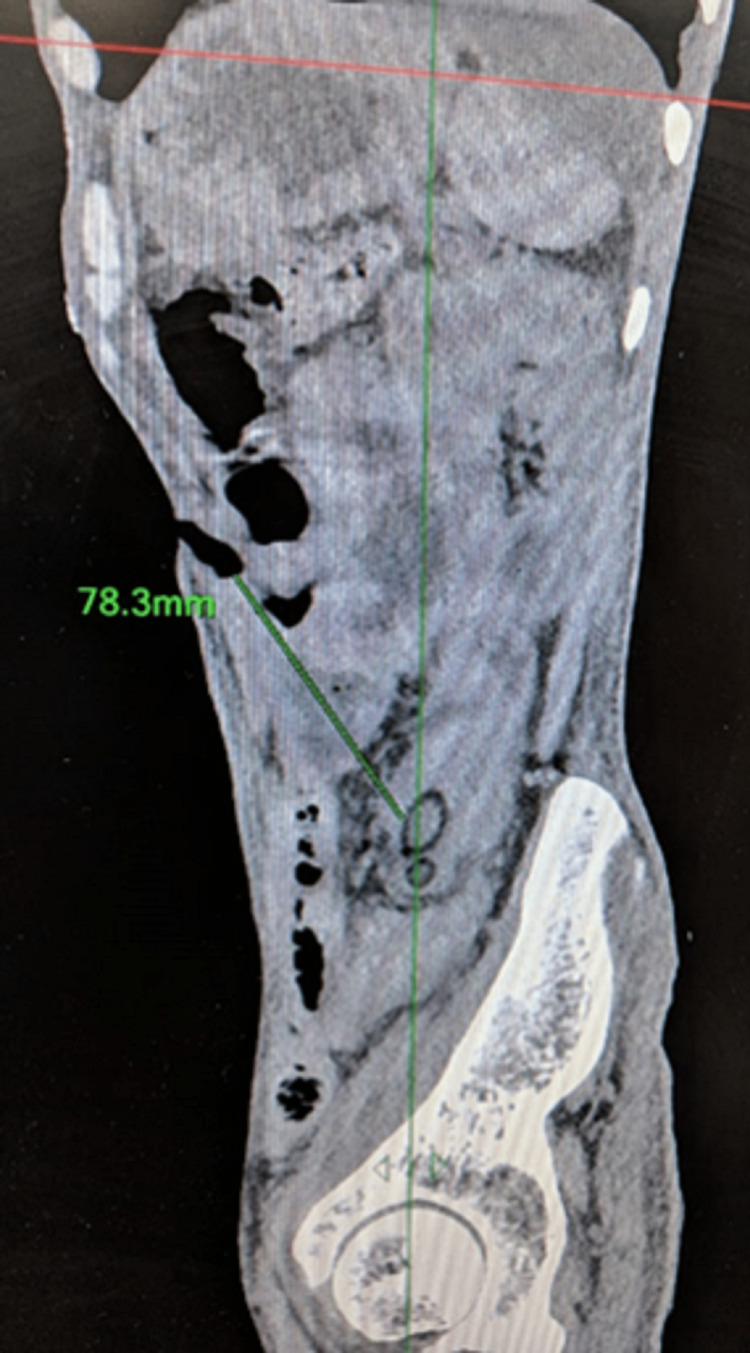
Contrast-enhanced CT (sagittal section ) showing a 78.3 mm displacement of Foley from the jejunostomy site.
